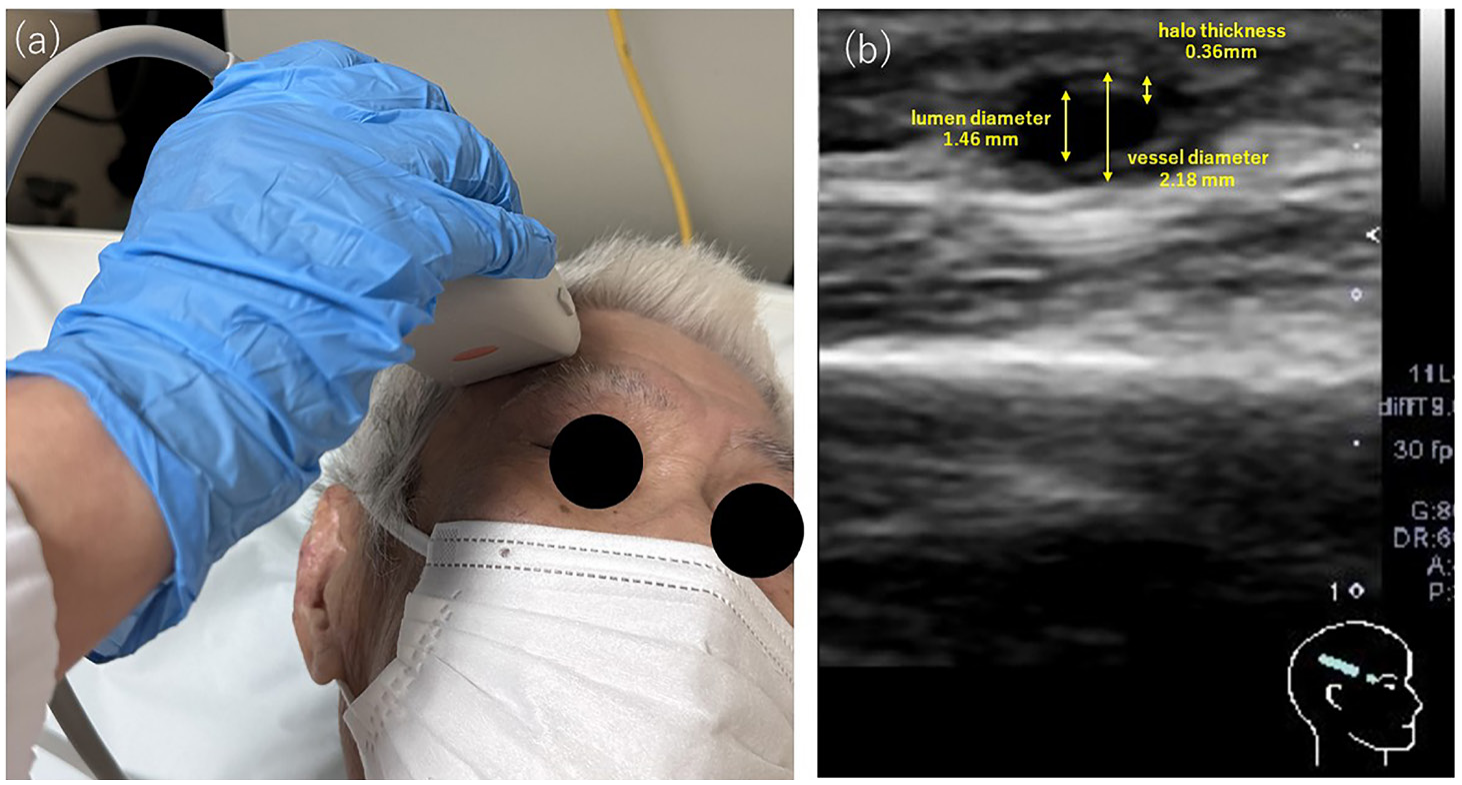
(a) Observations during temporal artery ultrasound examination. (b) Temporal artery ultrasonography revealed a perivascular halo sign.
